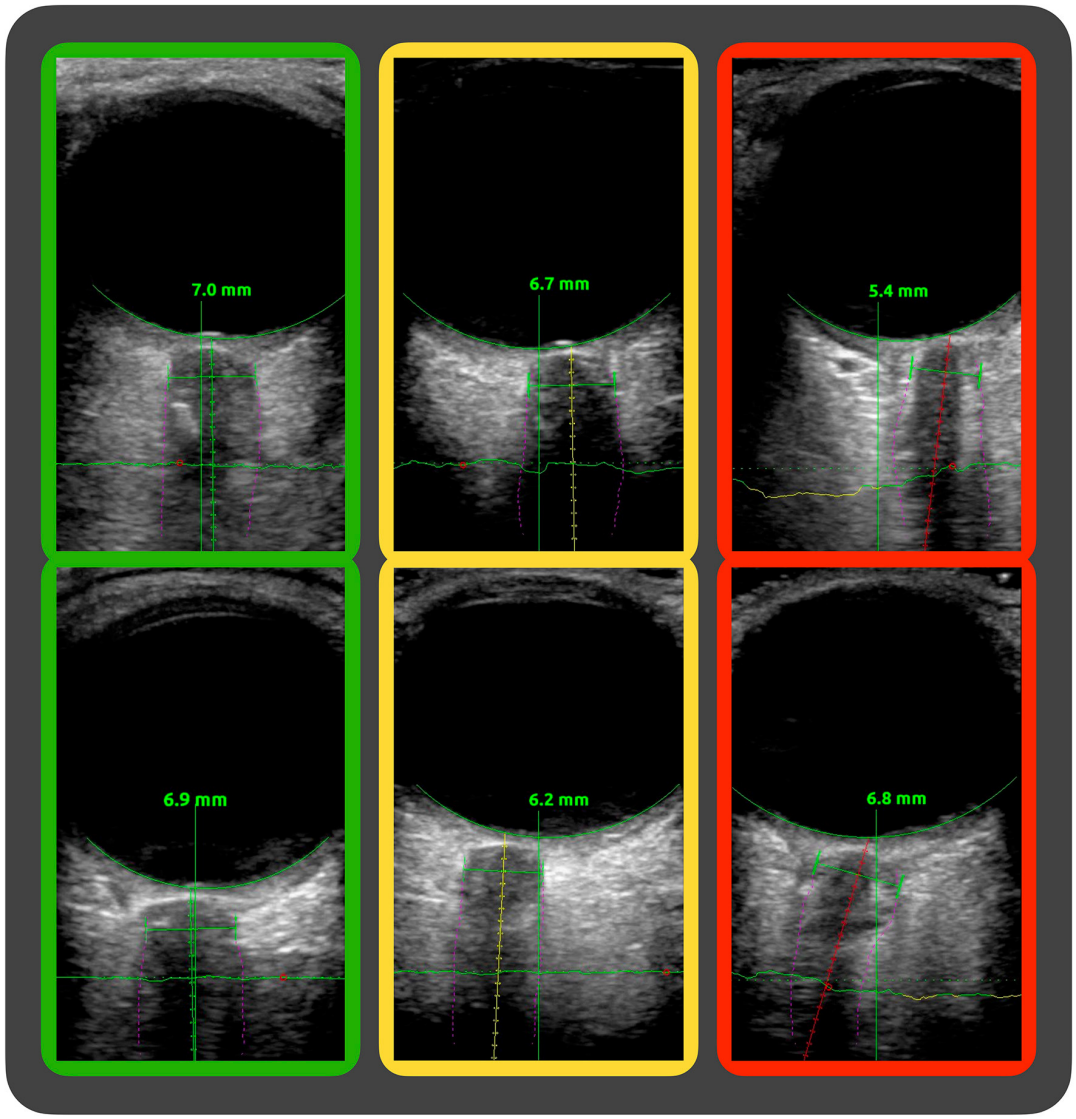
Image examples of the software's evaluation of axis alignment. Ultrasound images of the optic nerve sheath complex showing the software's segmentation (purple fine lines) of the nerve sheath and guidance system with differing evaluations of the axis alignment along the optic nerve. In the green, yellow, and red framed image, the software evaluation of axis alignment is good, intermediate, and poor, respectively.
